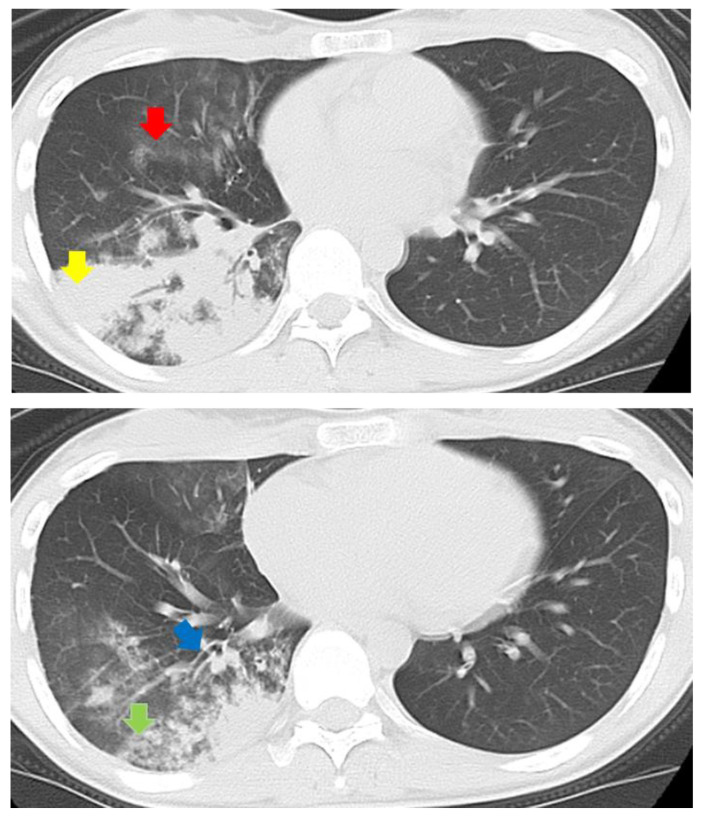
Chest CT of a 24-year-old Japanese woman with MPP showed various findings such as ground-glass attenuation (red arrow), consolidation (yellow arrow), centrilobular nodules (green arrow), and branchial wall thickening (blue arrow).
